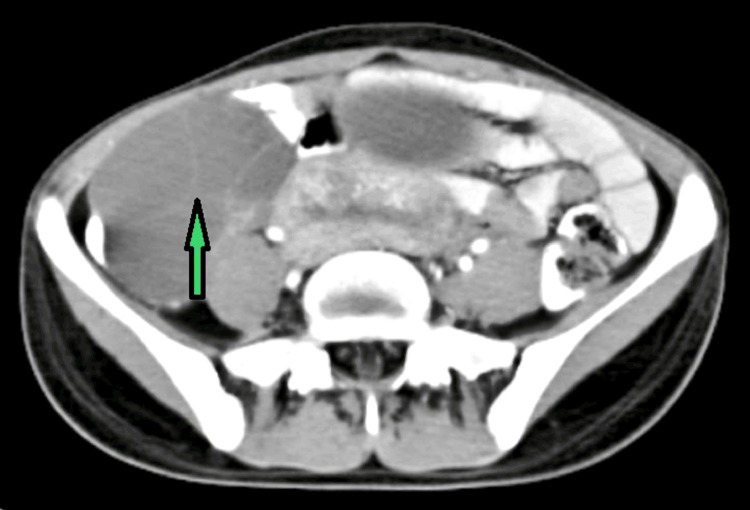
HRCT image showing bulky ovaries (arrow) HRCT: High-resolution computed tomography
